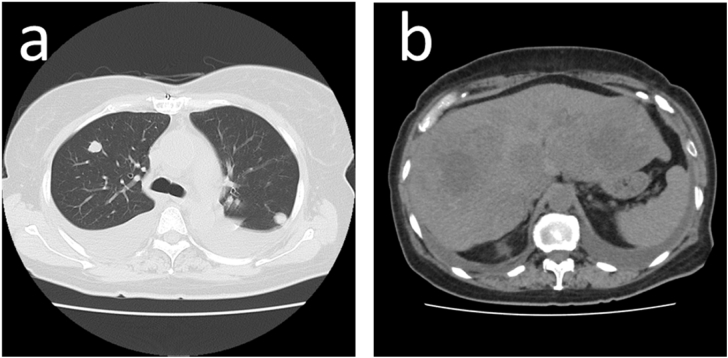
Computed tomography shows lung metastasis (a) and liver metastasis (b).
